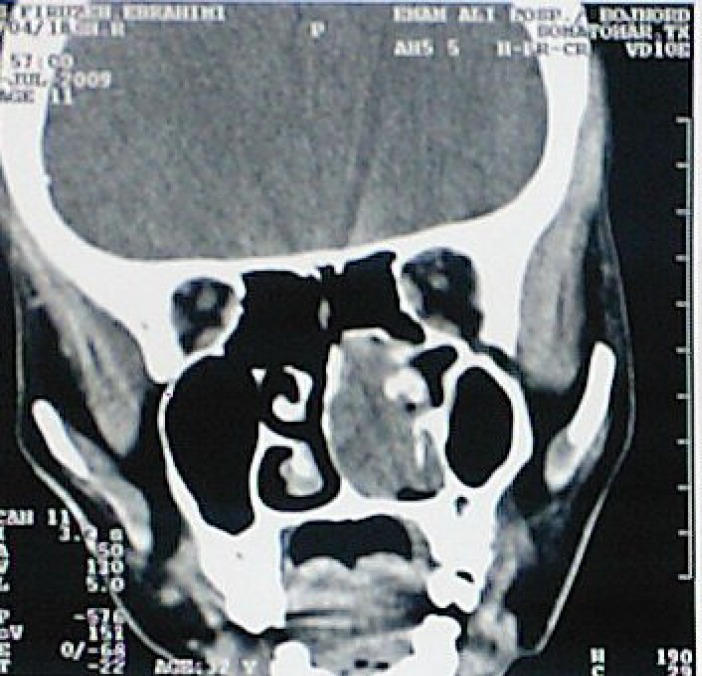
Paranasal sinus CT scan shows Intranasal mass lesion
